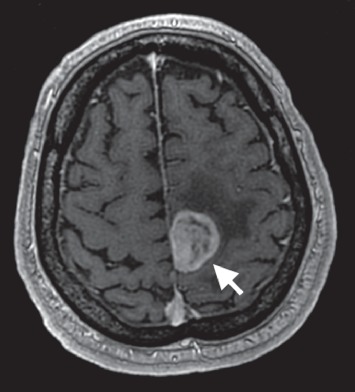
T1 postgadolinium MRI brain with axial view. This reveals a peripherally enhancing and centrally necrotic appearing mass in the left frontoparietal lobe with surrounding edema.
